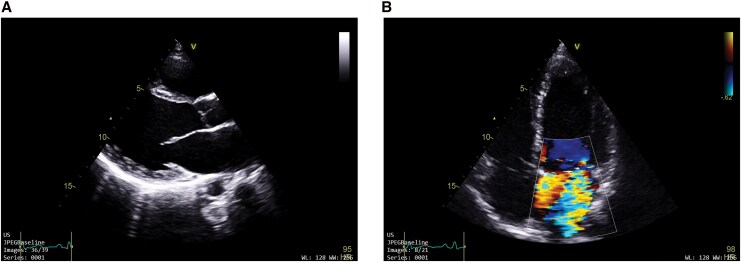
(*A*) (parasternal long axis view) follow-up echocardiography showed resolution of mass. (*B*) (Apical 4 chamber view) Follow-up echocardiography showed severe mitral regurgitation.
